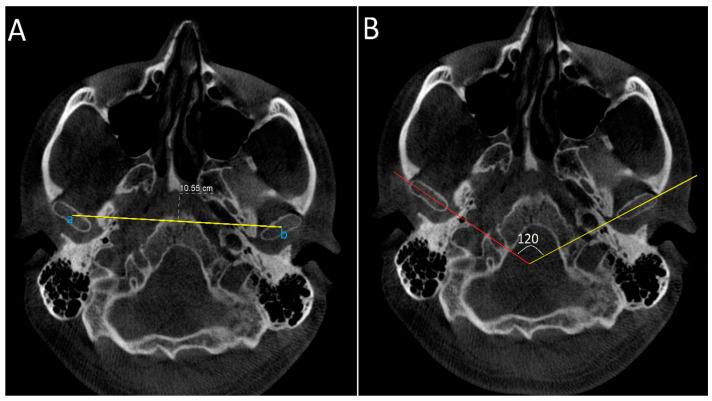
Axial CBCT images of a patient showing the measurement of the axial intercondylar distance and angle. In image (A), the axial intercondylar distance equals the yellow line joining point (a), equivalent to the centre of the right condyle, and point (b), equivalent to centre of the left condyle. In Image (B), the axial intercondylar angle is the angle between the lines joining the medial and lateral pole of the right condyle (red line) and the line joining the medial and lateral poles of the left condyle (yellow line).
